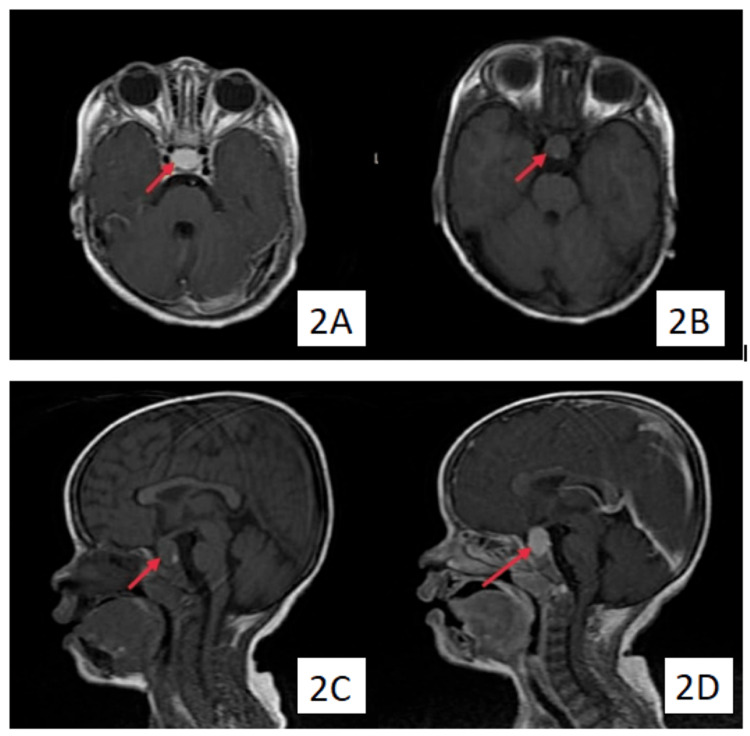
(A) Axial T1-weighted (post-contrast), (B) axial T1-weighted (pre-contrast), (C) coronal T1-weighted (pre-contrast), and (D) coronal T1-weighted (post-contrast) sections showing a homogenously enhancing extra-axial mass lesion in the sellar and suprasellar lesion
